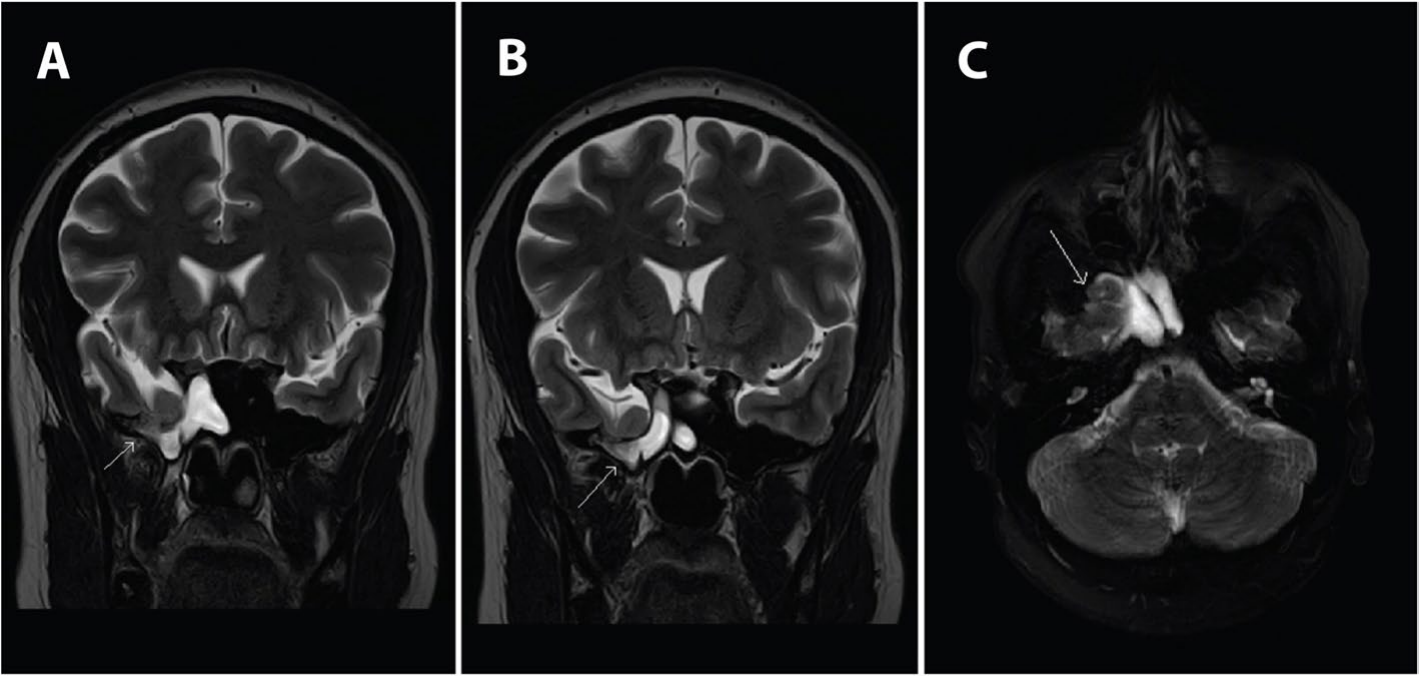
A & B Adjacent slices of a coronal T2-weight MRI showing a focal defect in the floor of the middle cranial fossa and a meningoencephalocele continuous with defect. C T2 axial view of the same findings
